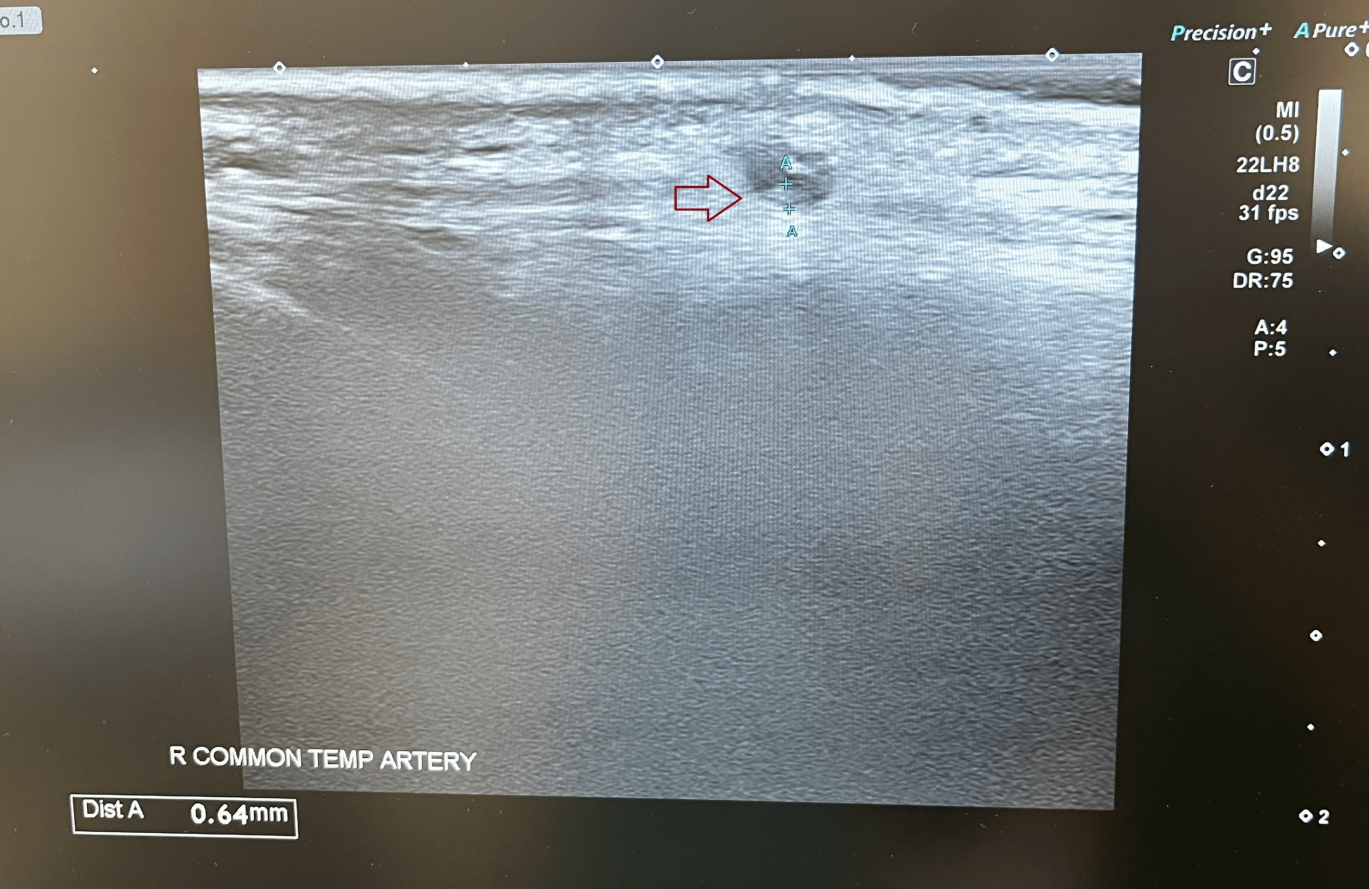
Temporal artery ultrasound showing intima thickness of 0.64 mm and minimal blood flow through it. Arrow points out the temporal artery blood vessel with an abnormal thickness >0.3 mm, indicative of vasculitis, also known as halo sign.
